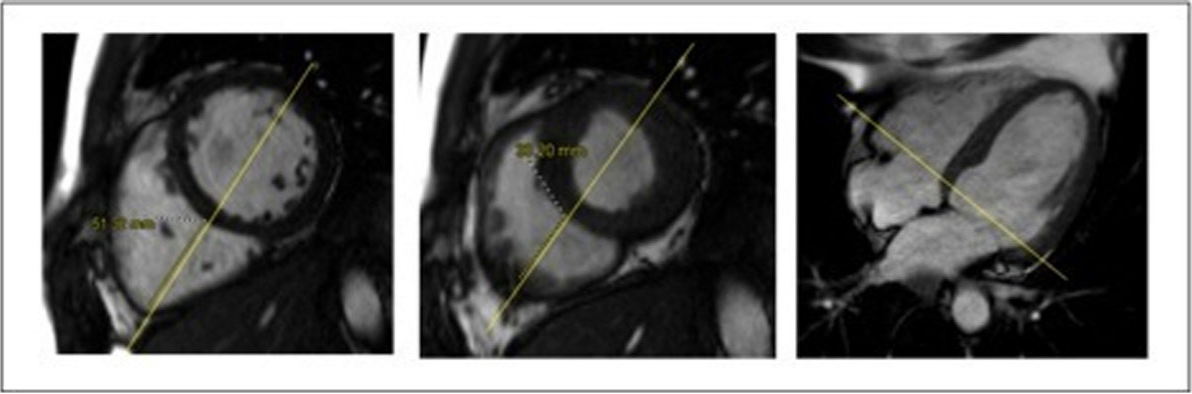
# Accuracy of a new method for semi-quantitative assessment of right ventricular ejection fraction by cardiac MRI: right ventricular fractional diameter changes
